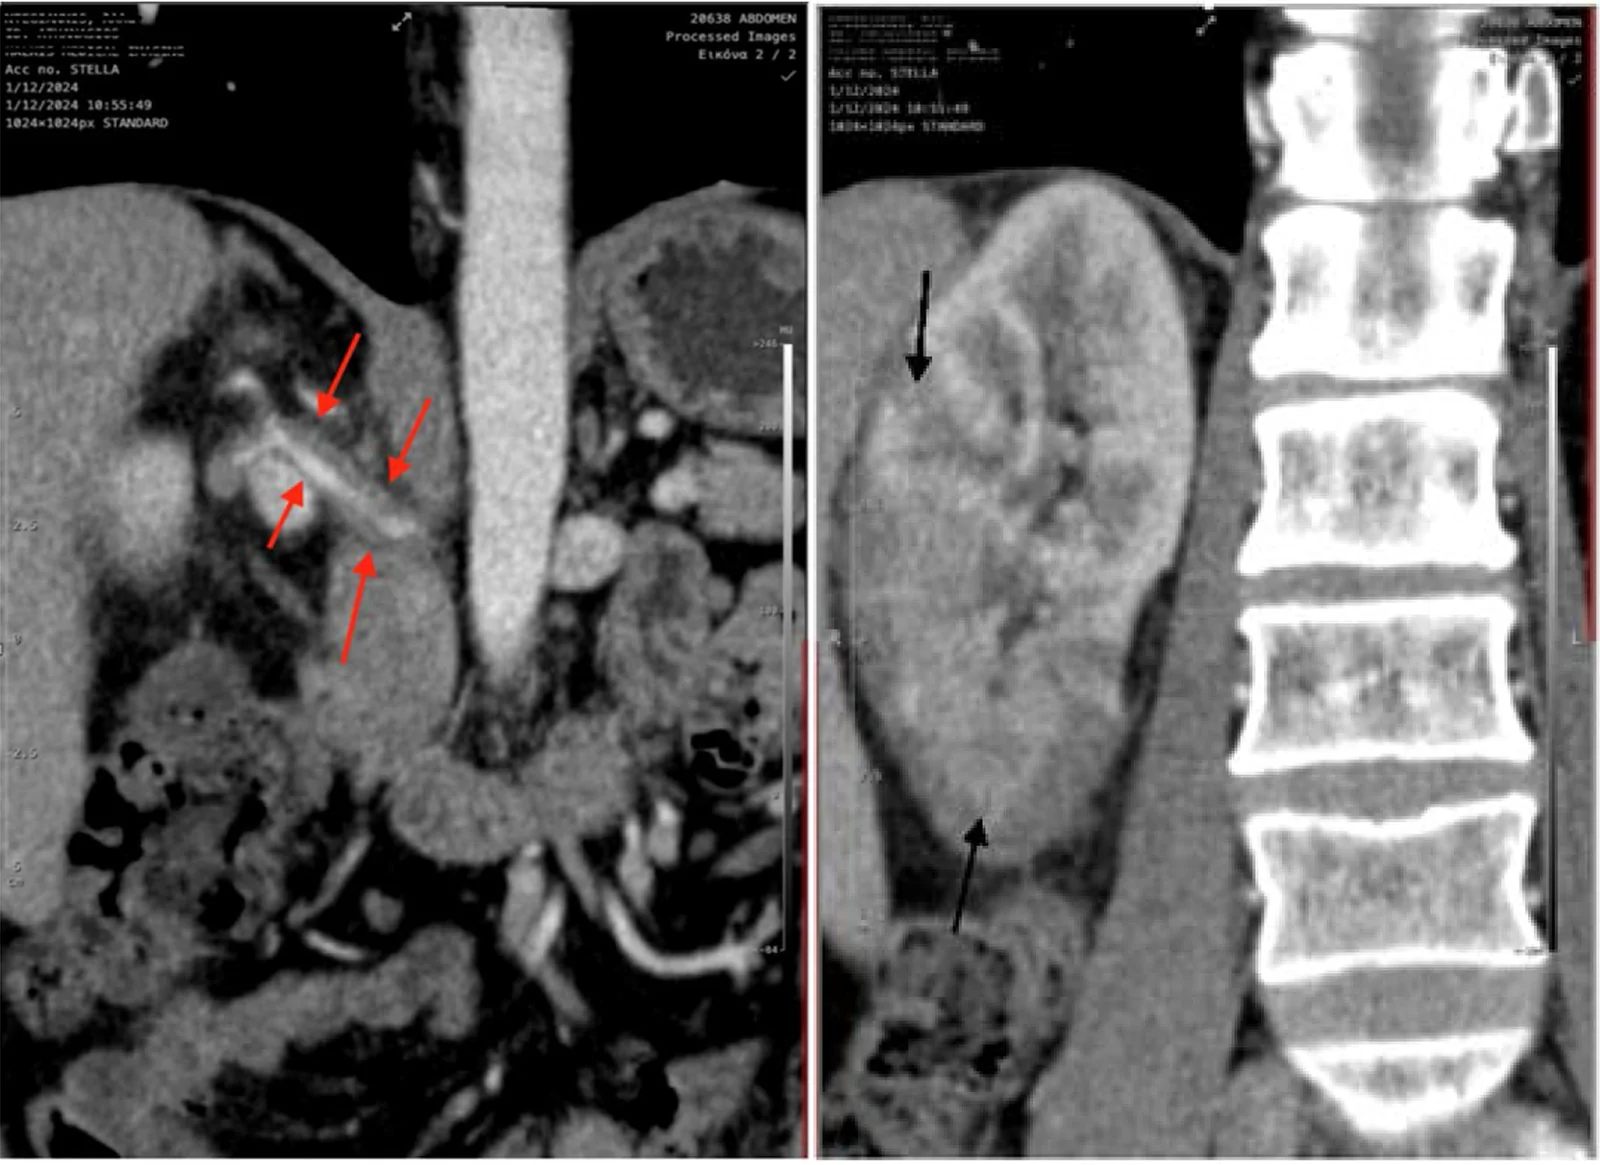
CT scan of the retroperitoneum showing right renal artery vasculitis with vessel wall thickening (red arrows) and multiple concomitant renal parenchymal infarcts (black arrows).
